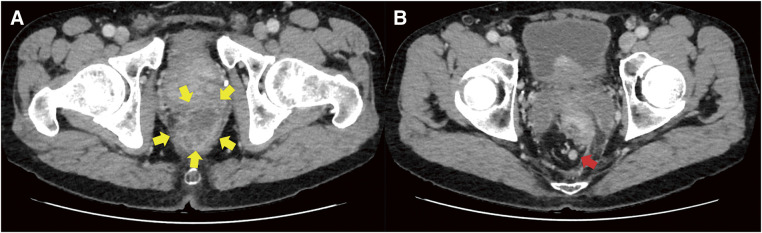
Enhanced CT. (A) A 48-mm mass with contrast enhancement was shown on the oral side of the ileoanal anastomosis in the ileal pouch (yellow arrow). (B) Enlarged lymph nodes were detected in the ileal mesentery (red arrow). CT, computed tomography
